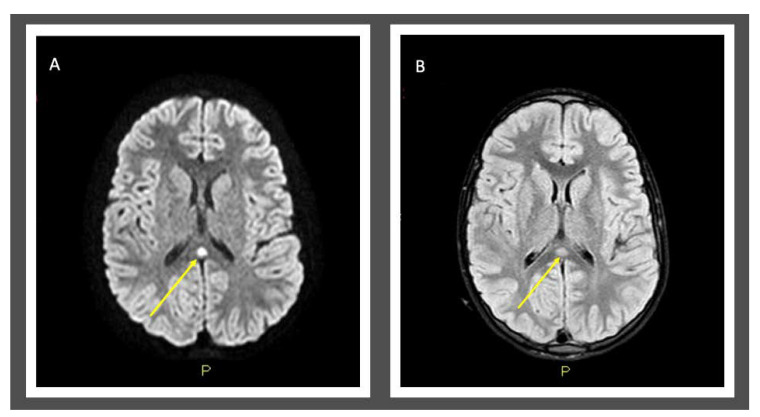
MRI of the brain (performed at day-4 of illness) showed splenial signal hyperintensity on T2-weighted sequence (A) and splenial restricted diffusion on diffusion weighted imaging (B).
